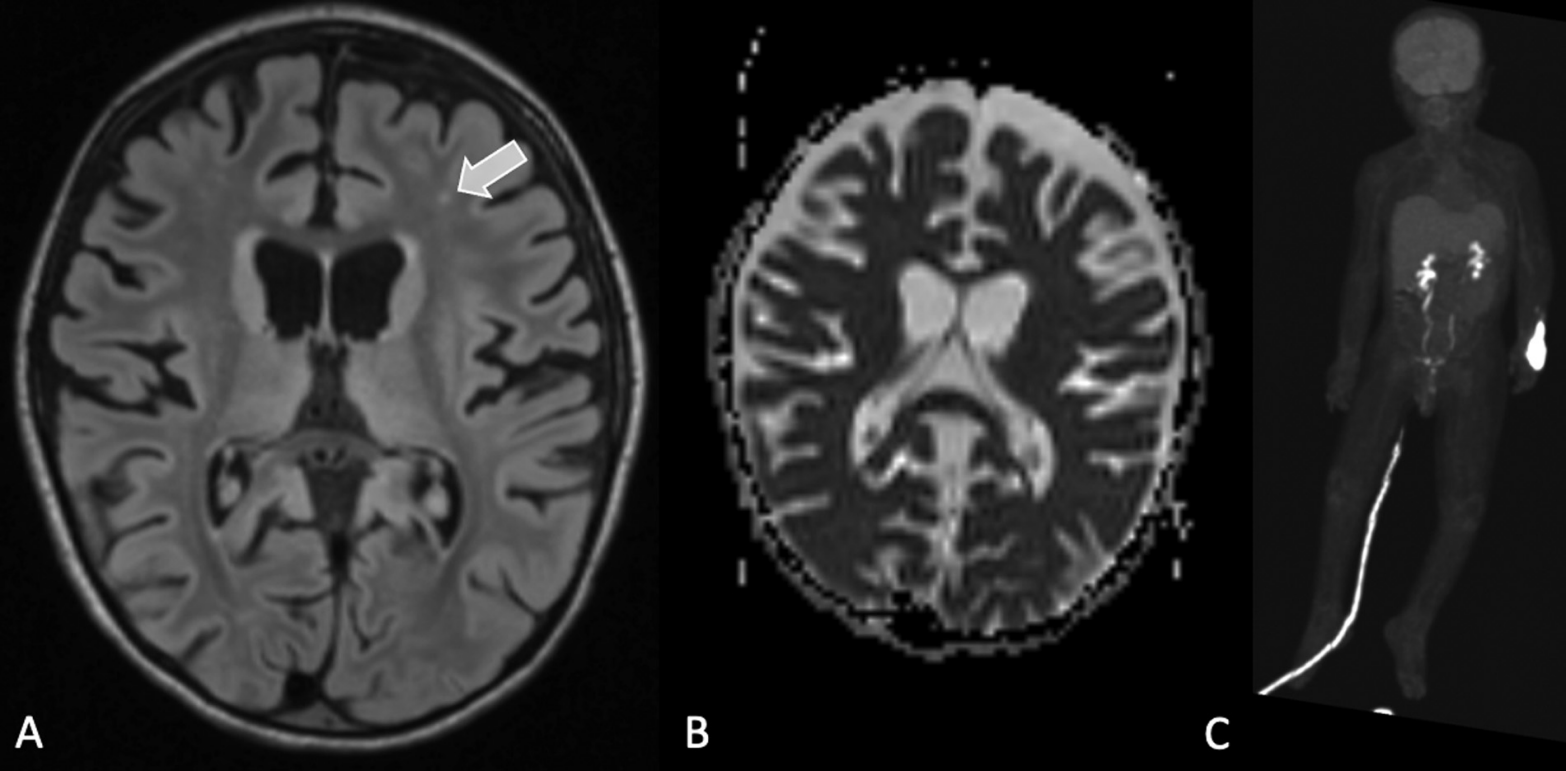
A 3-year-old child with refractory status epilepticus. MRI brain demonstrates parenchymal volume loss and white matter FLAIR hyperintense foci (
